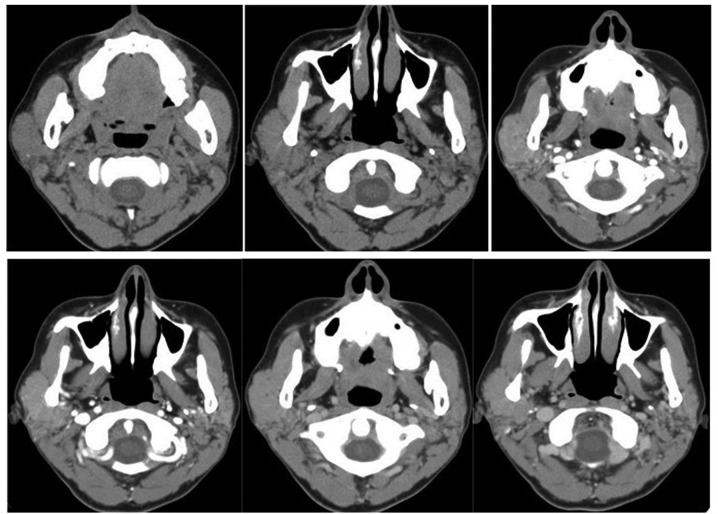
CT of a 58-year-old female patient showing two foci on the bilateral parotid gland, forcing the right side into an irregular shape, with slight lobulation. The necrosis inside the foci was more apparent following CT enhancement. The shape of the vessels was enhanced on the enhancement CT scan and the margin was unclear between the focus and the mass. CT, computed tomography.
